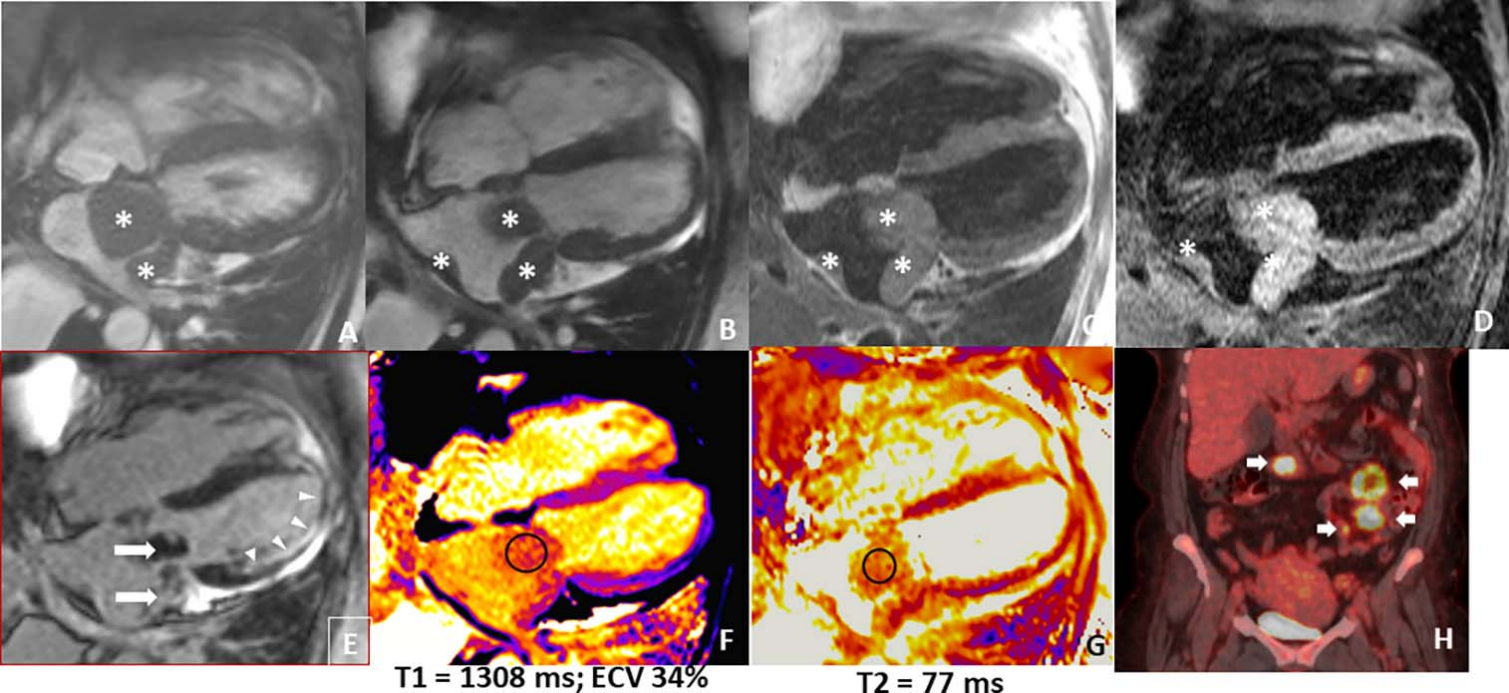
(A, B) Steady-state free precession magnetic resonance imaging sequence shows multiple left atrial masses, with the largest mass in relation to the fossa ovalis and the mitral valve. (C) Isointense signal to the myocardium on T1-weighted imaging. (D) Hyperintense signal to the myocardium on T2-weighted imaging. (A, B, C, D) Asterisks show the locations of the left atrial masses. (E) Heterogenous late gadolinium enhancement (arrows) and incidental infarct pattern at the lateral and apical segments (arrowheads). (F, G) Parametric mapping with increased T1 signal suggests inflammation, and increased T2 signal is consistent with edema. The circles represent the relaxation time measurement in the tumor on T1- and T2-weighted imaging which demonstrates the high fluid and interstitial content within the tumor. (H) Positron emission tomography scan shows multiple hypermetabolic foci (arrows). ECV, extracellular volume; ms, milliseconds.
